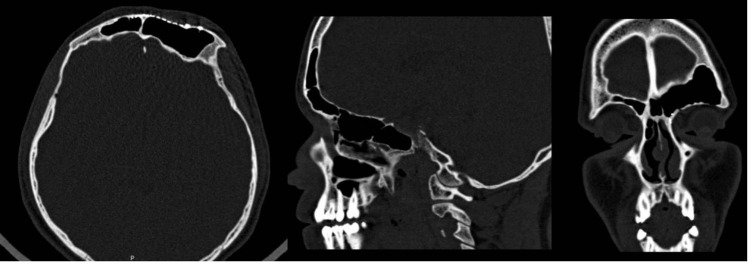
Postoperative CT scan Left frontal sinus osteoma in postoperative view.
